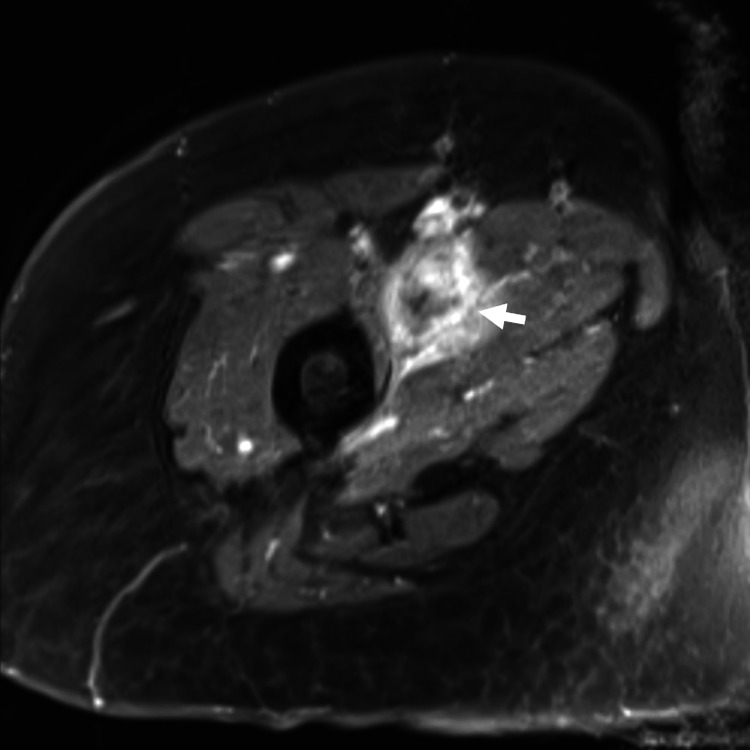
MRI of right thigh showed heterogeneously enhancing mass at the anteromedial aspect of the right thigh as shown on the white arrow.
